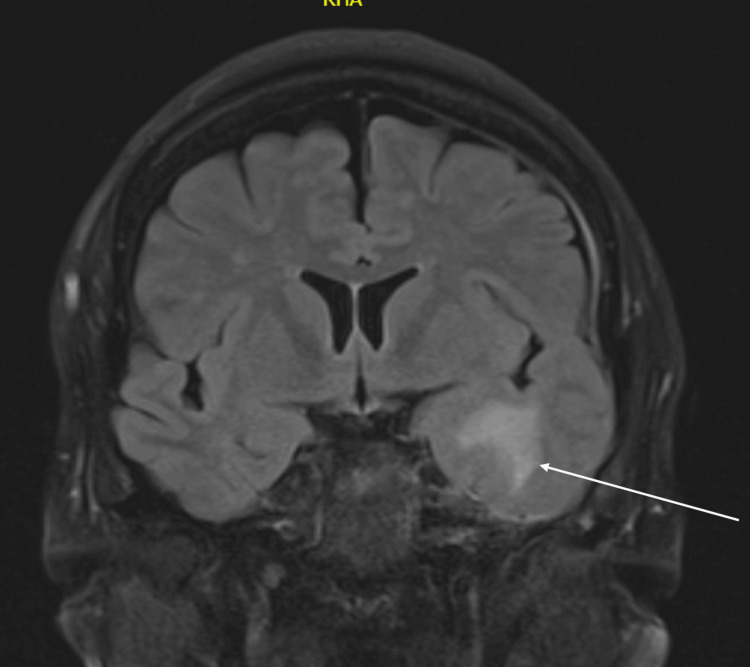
Fluid-attenuated inversion recovery (FLAIR) sequence magnetic resonance imaging (MRI). FLAIR sequence MRI showing gliotic changes in the left temporal lobe (white arrow). The annotated region corresponds to postoperative gliosis at the prior surgical site.
